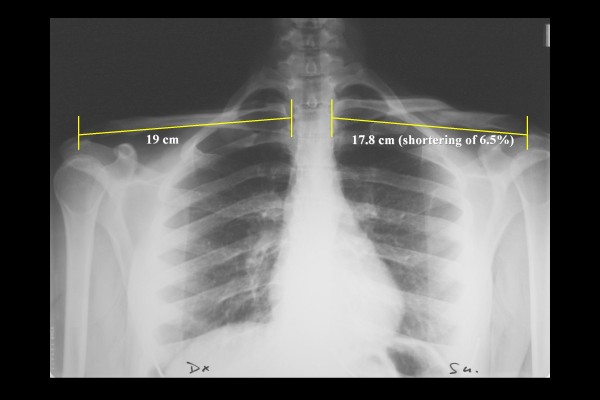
Radiographic image of the clavicle of a satisfied patient after conservative treatment of a diaphyseal clavicle fracture. The observed shortening was 12 mm, or 6.5%; the Constant score was 80.
